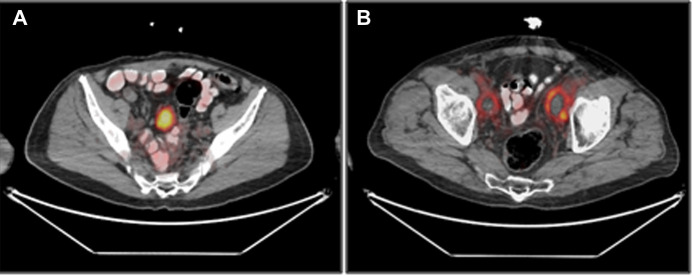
Soft tissue uptake of [^18^F]PSMA-1007, 1 h after injection. (A) uptake in a pelvic mass, subsequently identified as a desmoid tumor (patient #9). (B) Uptake in bilateral pelvic lymphoceles (patient #13).
